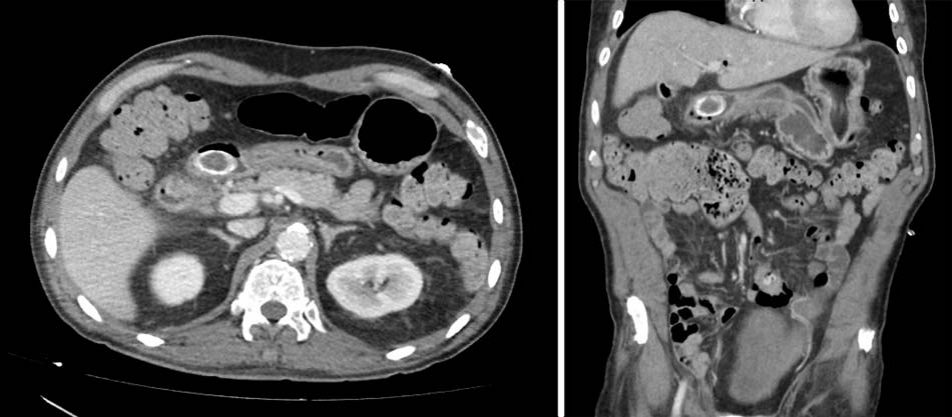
Contrast-enhanced CT. Axial and coronal views of a 4 cm stone in the duodenal bulb, gastric dilation, and pneumobilia in the operative patient.
